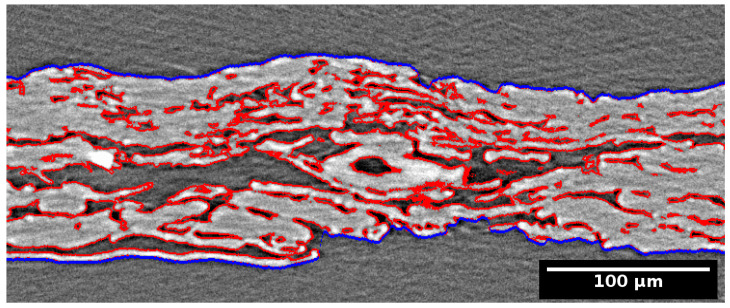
Visualisation of the X-ray microtomograph (CT) image of a sample pressed at 190 °C temperature (grayscale), edges of segmented regions (red) and surfaces of the sheet (blue).
